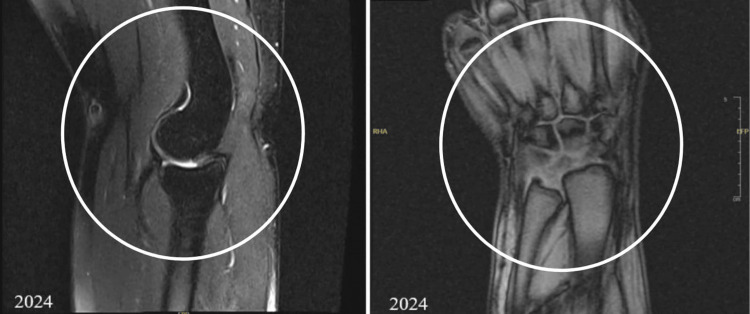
Right elbow and wrist MRI, elbow MRI showed no joint effusion or erosions. Mild sprain at the ulnar collateral ligament and the fibers are intact. The wrist MRI showed a trace amount of fluid in the distal radial ulnar joint with abnormal signal intensity at the radial side of the triangular fibrocartilage disc which may suggest a tear and there was negative ulnar variance.
